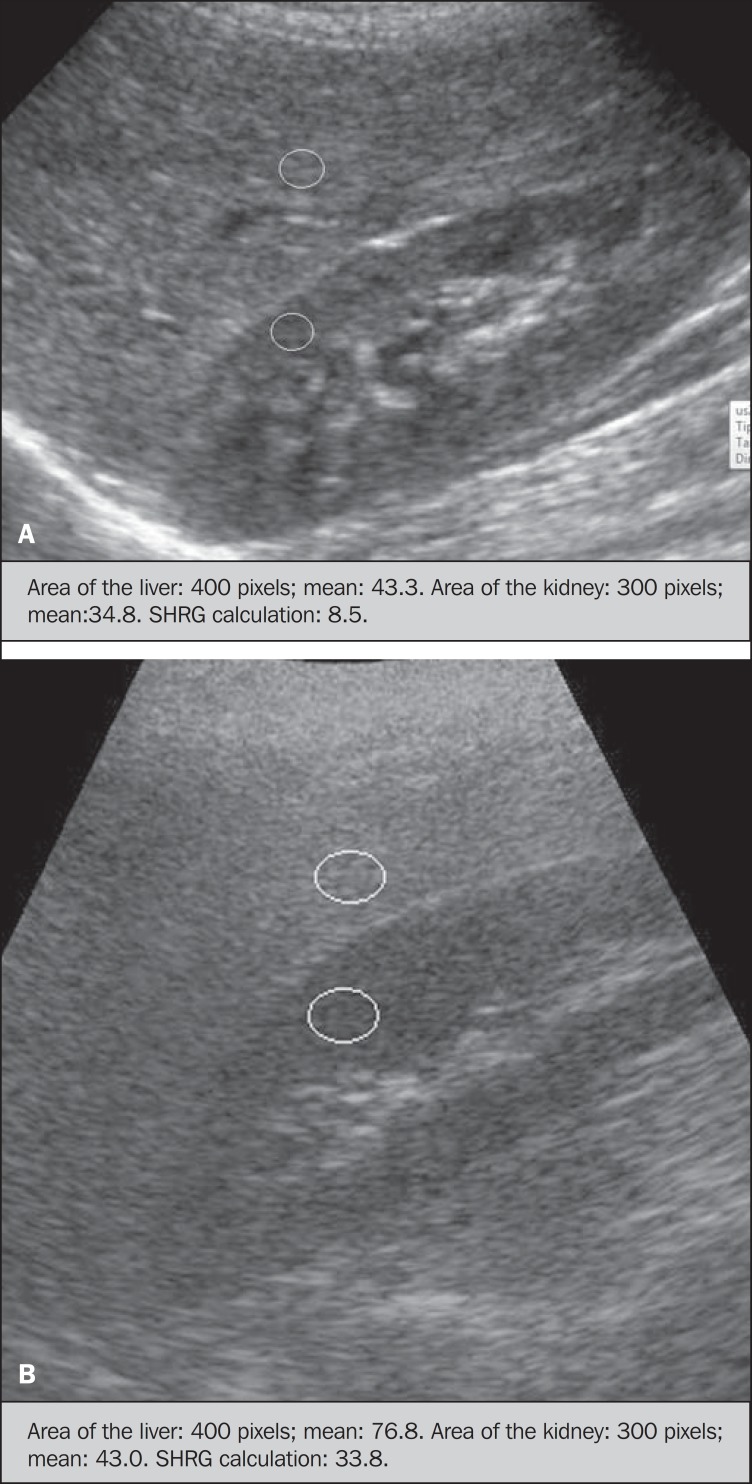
Sonographic images demonstrating the regions of interest. A: Liver
